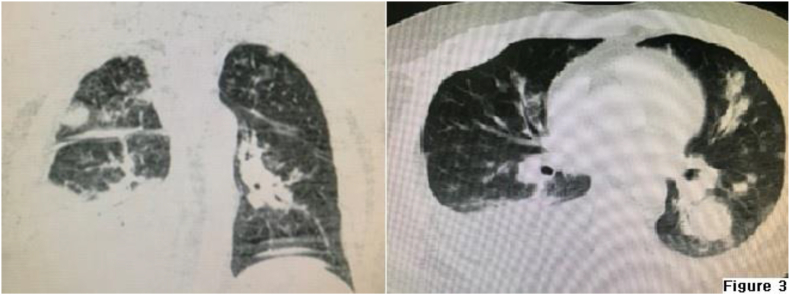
Lung metastasis in Chest CT scan, one month after the surgery.
